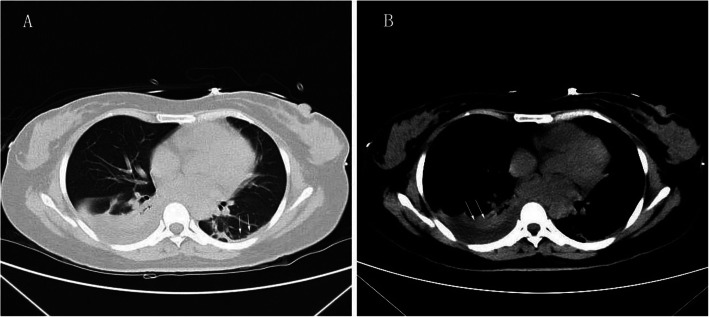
Lung CT scan showing left pleural thickening and right pleural effusion
